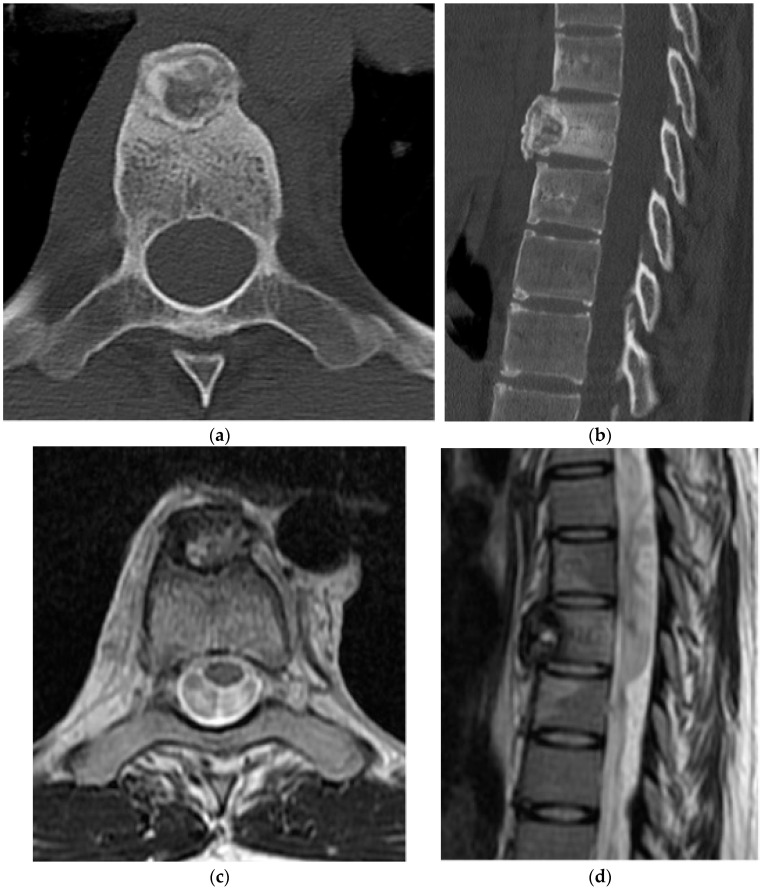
Osteoblastoma. (a) Axial and (b) sagittal CT bone window, expansile lesion at the anterior margin of T10 with a narrow zone of transition and a central nidus. The lesion measures greater than 1.5 cm, distinguishing it from an osteoid osteoma. Increased sclerosis in the involved vertebral body is due to chronic inflammation. (c) Axial and (d) sagittal T2 sequences, predominantly T2 hypointense lesion with cortical thickening. There is prominent perilesional edema extending into the adjacent vertebral bodies and the paravertebral soft tissue. This is an atypical location for an osteoblastoma as most are found in the posterior elements (Figure 4).
